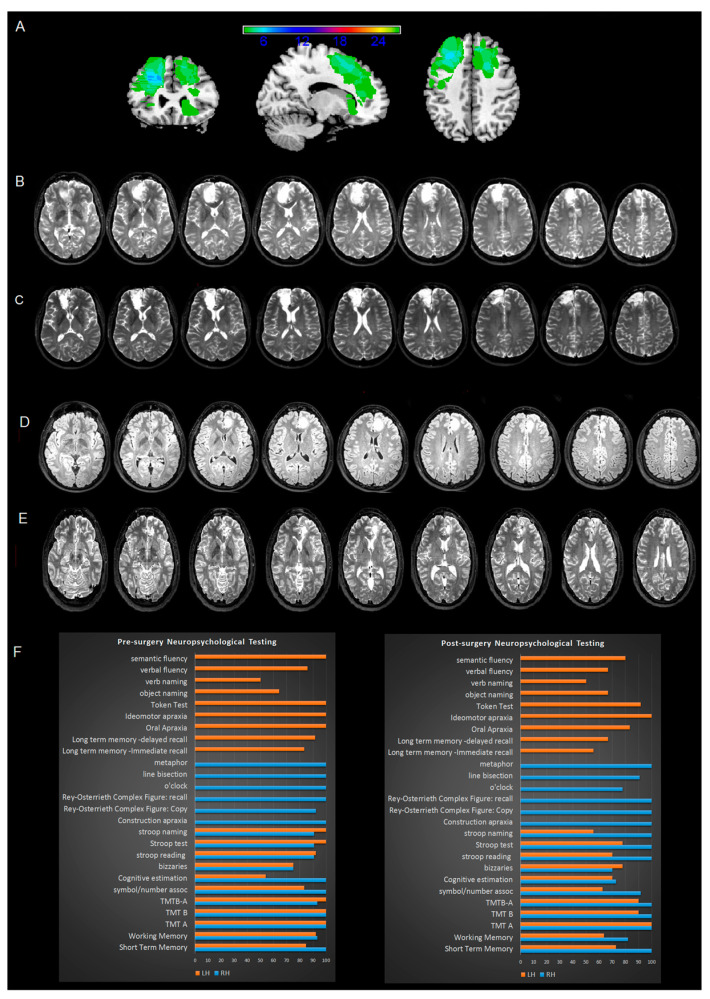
Patients’ lesion volumes of interest (VOIs) overlay (A) in addition to pre-surgery (B,D) and post-surgery (C,E) T2 MRI images of two representative patients. In (F), the % of patients’ scoring within the normal range during the pre- and post-surgery neuropsychological performance is shown.
